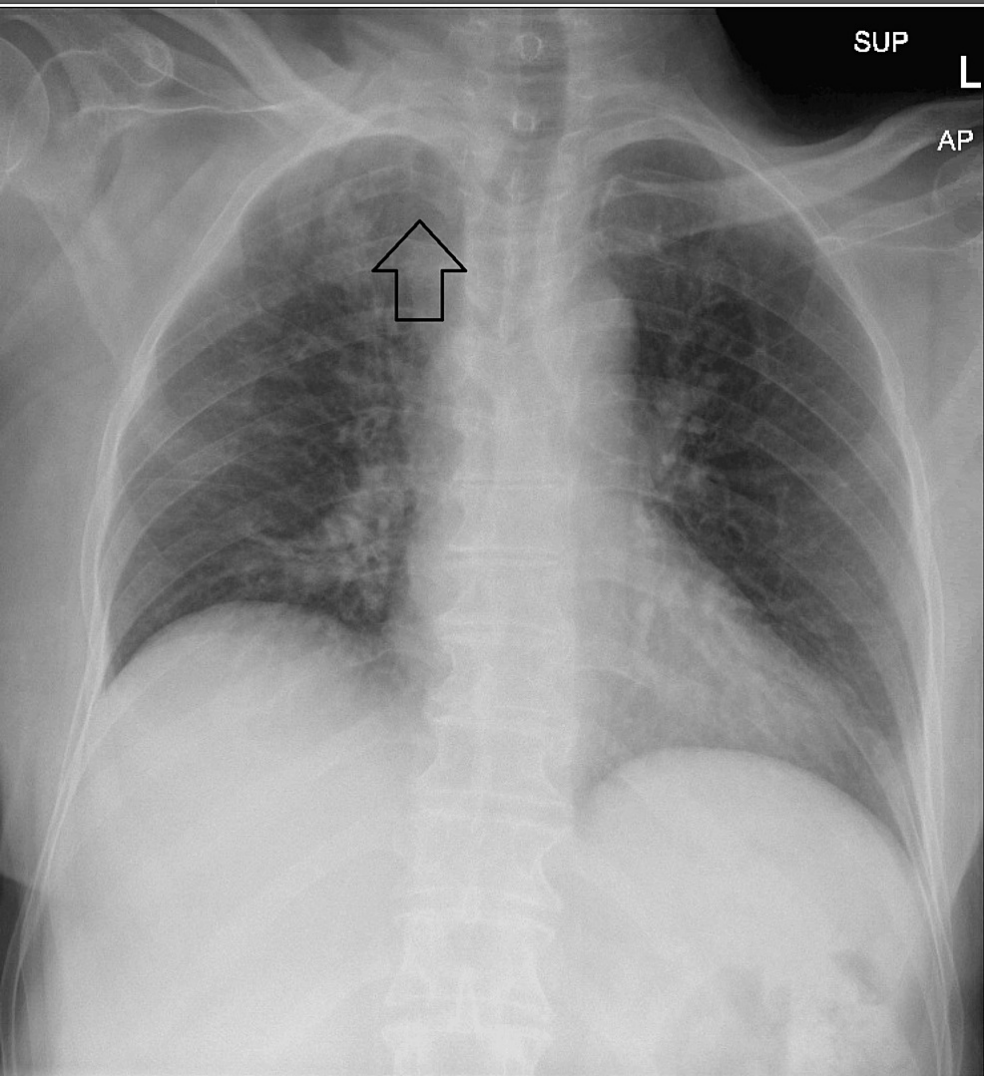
Postoperative X-ray showing the status post right medial clavicle resection
